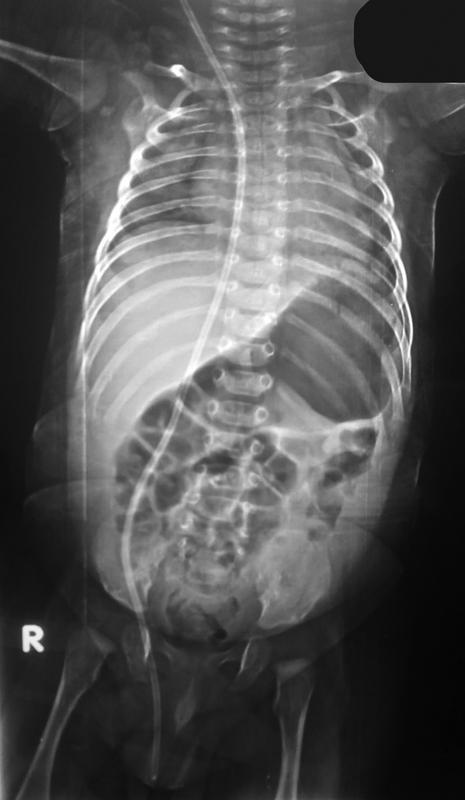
Plain X-ray of abdomen anteroposterior view, showing tip of peritoneal end of the shunt located in the right scrotum.
